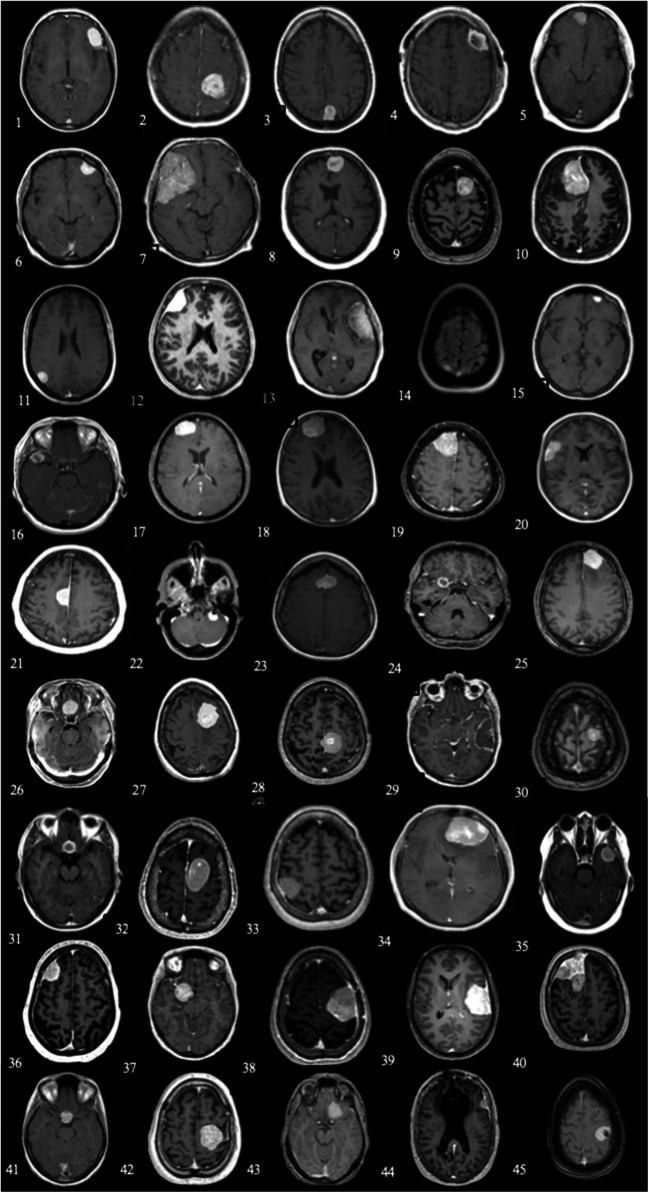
Representative T1-GD axial slice from the diagnostic MRI examination and cause of first radiological scan among asymptomatic patients. Left to right, patient 1 (upper left) through patient 45 (lower right). 1. Infarction^D^, 2. migraine, 3. idiopathic intracranial hypertension, 4. trauma, 5. depression, 6. vertigo*, 7. control of head/neck cancer*, 8. numbness in hand and arm*, 9. pulsating sensation in ear^S^, 10. fainting, 11. meningitis, 12. migraine*, 13. neck pain^S^, 14. examination before pregnancy*, 15. vertigo, 16. idiopathic intracranial hypertension*, 17. shaking in thumb caused by exertion, 18. control patient in study, 19. infarction*, 20. neck pain, 21. hallucinations, 22. vertigo*, 23. pain behind eye*^S^, 24. neck pain*, 25. infection of unspecified origin, 26. control of head/neck cancer*, 27. trauma, 28. trauma^D^, 29. control of head/neck cancer, 30. migraine*, 31. migraine*, 32. sepsis*^D^, 33. neck pain*, 34. examination of vestibula schwannoma, 35. neck pain*, 36. periorbital lipoma*, 37. trauma*^D^, 38. transient hearing loss*, 39. migraine*, 40. unspecified symptoms from ear*, 41. meningitis, 42. transient hearing loss, 43. control head/neck cancer*, 44. follow-up exam oligodendroglioma*, 45. fatigue*. The asterisk indicates growth prior to surgery. D indicates new onset deficit postoperatively. S indicates new onset seizure postoperatively
